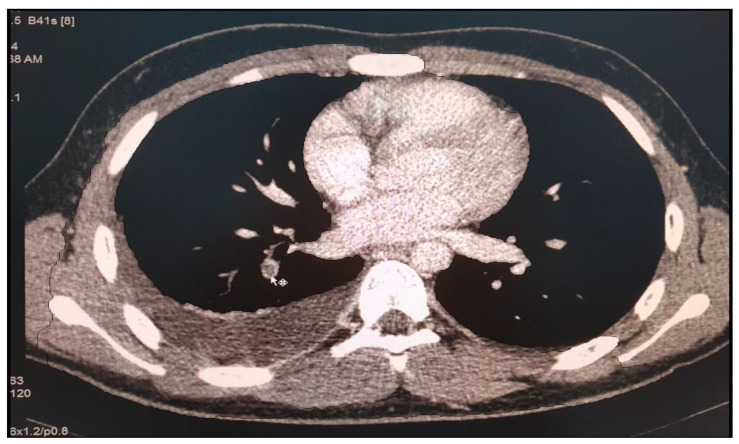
CT scan, appearance of right pulmonary artery segmental branch thrombosis, pulmonary embolism and right secondary pleurisy (personal collection Nephrology Department).
